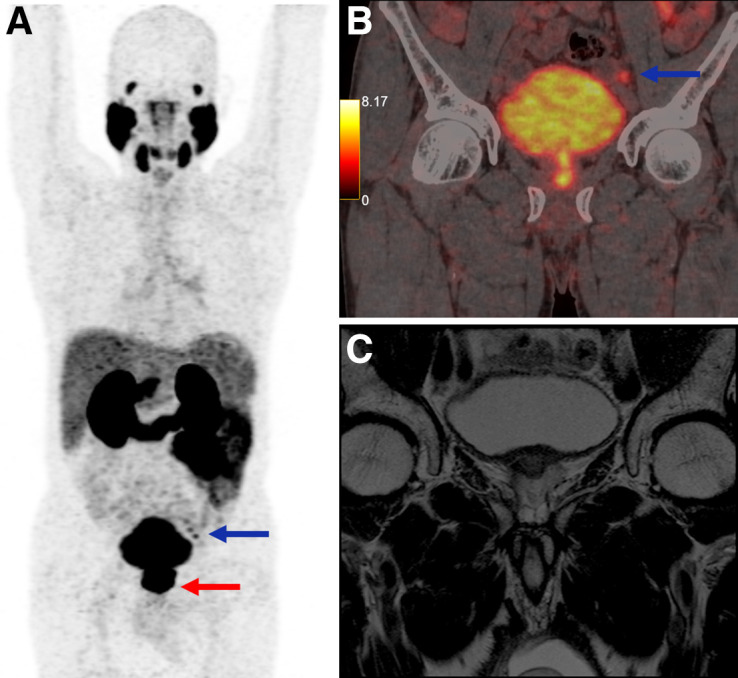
A 72-y-old man presenting for initial staging of PCa. (A) ^68^Ga-PSMA-11 PET/CT maximum-intensity projection shows PSMA-expressing lesion involving prostate (red arrow), with PSMA avidity in left external iliac lymph nodes (blue arrow). (B) PET/CT coronal image shows 1 lymph node involved (blue arrow). (C) However, MRI at same topography shows no lymph node involvement. Pathology analysis confirmed ^68^Ga-PSMA-11 PET/CT–positive result.
